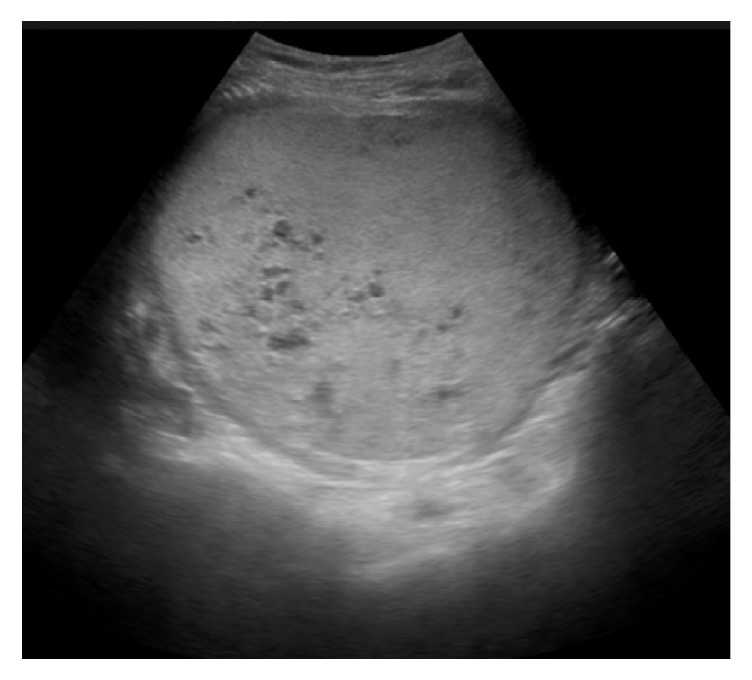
A pelvic ultrasound showing a large intrauterine heterogeneous mass that includes multiple discrete anechoic spaces consistent with complete mole.
